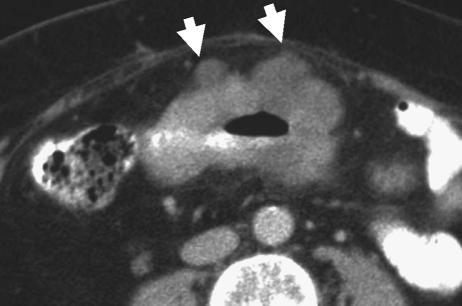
Preoperative CT scan showing a fungating tumour of the transverse colon. The anterior colonic wall is distorted by tumour. As there is minimal pericolic fat and the colon is peritonealised at this location, there is a very high probability that the tumour will be stage T4. Pathological staging was pT4pN1.
